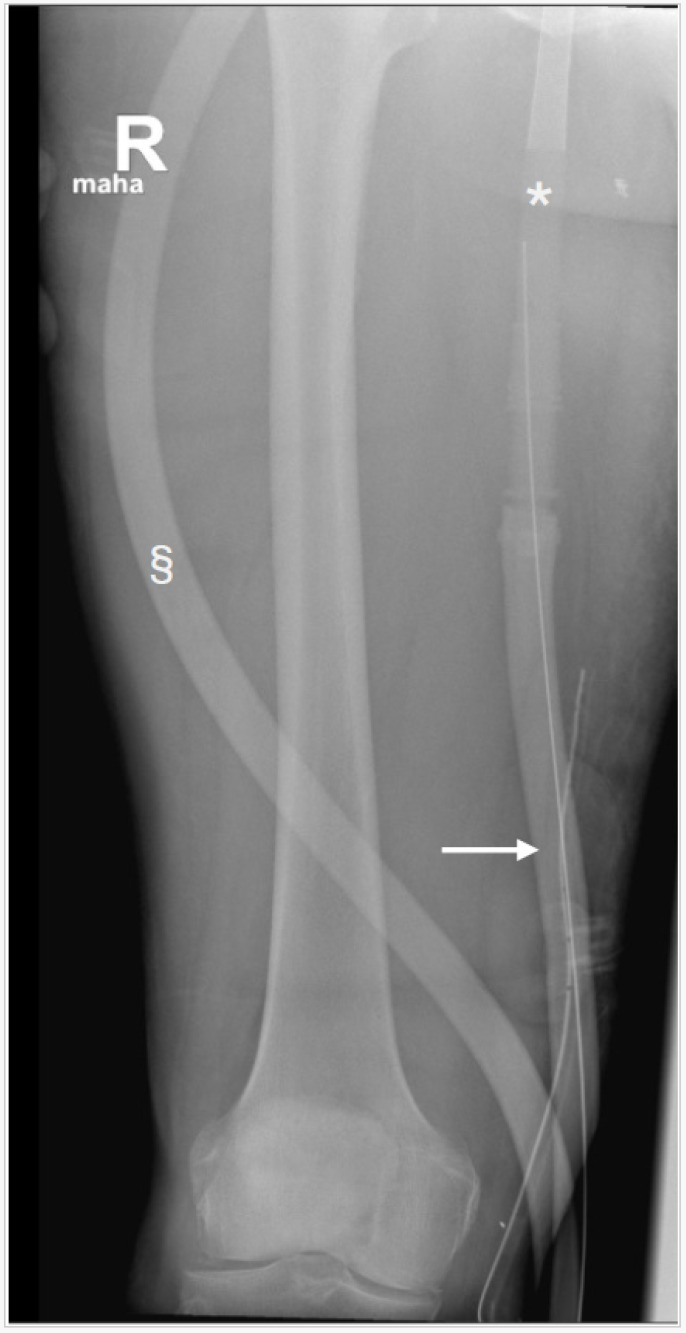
The image was obtained from a patient treated with veno-arterial extracorporeal membrane oxygenation (ECMO) for cardiogenic shock after ST-elevation myocardial infarction and acute coronary artery bypass surgery. Extracorporeal membrane oxygenation (ECMO) is increasingly used in patients with both, respiratory and cardiac failure. For patients with significantly impaired cardiac function, a veno-arterial configuration of ECMO-cannulas is necessary [1]. In the above patient, postoperatively, a second central venous catheter for continuous veno-venous hemofiltration was inserted ultrasound-guided via the right jugular vein using Seldinger’s technique. After puncture of the vein, the guidewire was inserted over the needle with another sonographic position check. During this procedure, the guidewire was aspirated into the venous system. On a consecutive chest X-ray, the lost guidewire was not visible. However, this X-ray of the right thigh indicated aspiration of the guidewire (→) by the venous ECMO cannula (*). § indicates the arterial ECMO circuit tubing. In the aftermath, the guidewire migrated into the venous ECMO tubing until the tip of the guidewire was withheld by the ECMO membrane. ECMO blood flow and function were not affected by the foreign material in the ECMO circuit. The guidewire was easily removed with an exchange of the ECMO system. While insertion of a central venous catheter (CVC) is a frequent procedure in critically ill patients, CVC placement itself is associated with several, mostly iatrogenic, complications [2]. Of those, intravascular retention of the guidewire used for CVC placement with Seldinger’s technique is a very rare but always avoidable complication. Loss of a guidewire in the vasculature must never occur. Because the venous drainage cannula of an ECMO circuit generates significant negative pressures, special vigilance to prevent venous suction of an unsecured guidewire is required in patients treated with ECMO. Adherence to standardized protocols and operating procedures is a measure used to prevent any loss of a guidewire.
